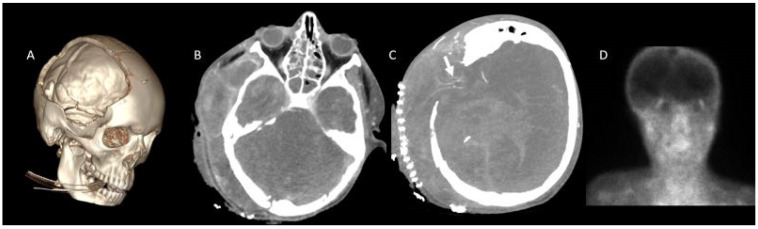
CTA in a 13-year-old boy with clinically confirmed brain death. (A), Craniectomy defect is visualized in VR images. (B), A lack of opacification is observed in the SPV and ICV during the venous phase. (C), However, persistent opacification of the right MCA-M4 segment is noted (white arrow). (D), Brain perfusion was absent on scintigraphy, further supporting the diagnosis of brain death. CTA: CT angiography, MCA: Middle cerebral artery, ICV: Internal cerebral vein, SPV: Superior petrosal vein, VR: 3D volume-rendered.
